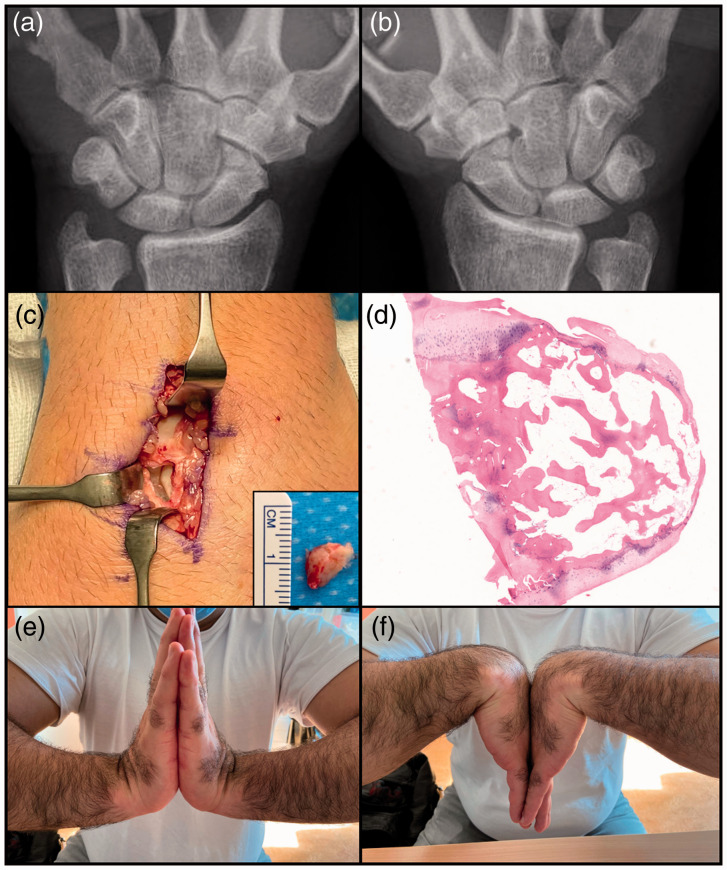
Patient 1. Posteroanterior radiographs of the left (a) and right wrists (b).
